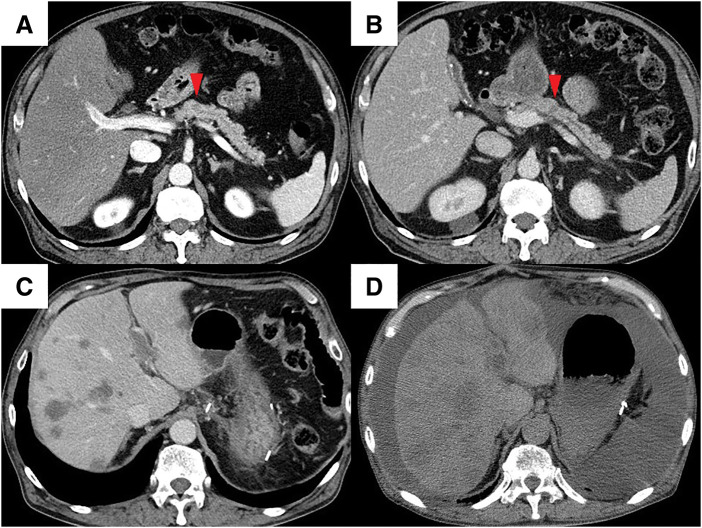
CT images of Case 2 (A) 12 mm hypovascular tumor observed in the pancreatic body (arrowhead) before neoadjuvant GS therapy. (B) Tumor remained 11 mm in diameter, with no change in size (arrowhead) after neoadjuvant GS therapy. (C) Multiple hepatic metastases and portal vein tumor thrombus were observed on POD 125. (D) Increased hepatic metastasis and ascites were shown on POD 176. GS, gemcitabine plus S-1
